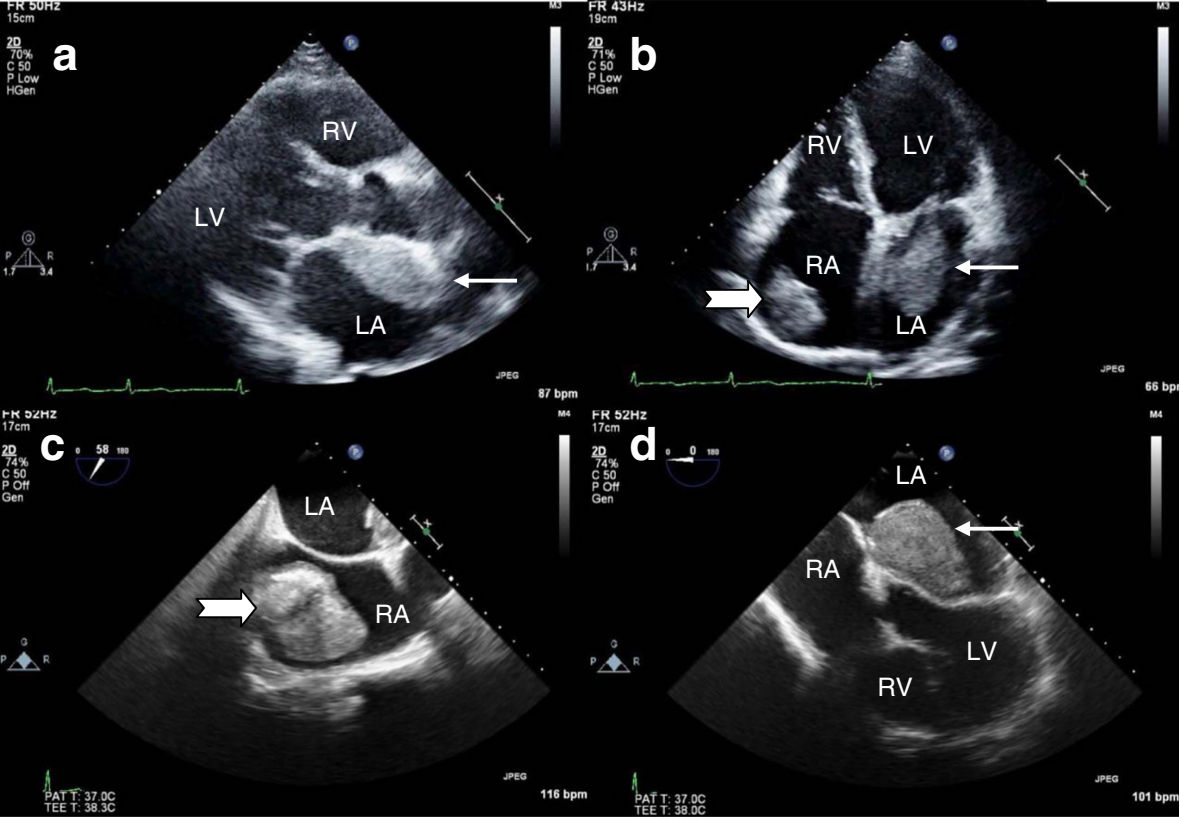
Images obtained by transthoracic echocardiography and transesophageal echocardiography. a, b Transthoracic echocardiography images. c, d Transesophageal echocardiography images. A large left atrial mass (*arrows*) adhering to interatrial septum in parasternal long axis (a) and apical four chamber view (b, d) and a giant right atrial mass (*notched arrows*) was seen in apical four chamber (b) and bicaval view (c). *LA* left atrium, *LV* left ventricle, *RA* right atrium, *RV* right ventricle
